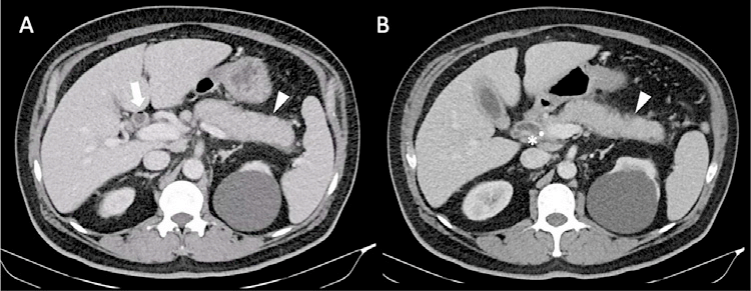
Axial CT images of the abdomen with diffuse enlargement of the pancreas, loss of clefts, and minimal peri-pancreatic stranding (white arrowhead). There is also thickening of the common bile duct wall with trace pneumobilia (white arrow) in setting of biliary stent placement (asterisk). Mild thickening of the gallbladder wall is also seen in (B) without cholelithiasis.
